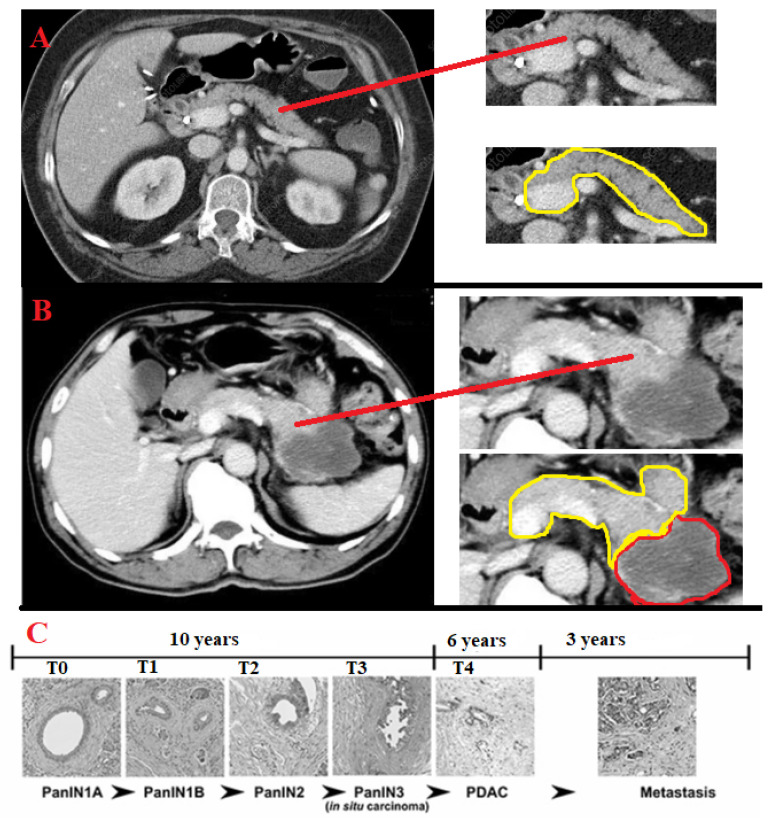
(A) A normal pancreas CT image; (B) PDAC at an advanced stage, with spreading to other organs; (C) Stages of PDAC development, which are represented as T0 to T4. The other stage, metastasis, is not represented because cancer at that point has spread to other organs surrounding the pancreas. PanIN (pancreatic intraepithelial neoplasia) with low-grade pancreatic intraepithelial neoplasia encompasses three older terms—PanIN-1A, PanIN-1B, and PanIN-2. These include Pancreatic Intraepithelial Neoplasia 1-A, Pancreatic Intraepithelial Neoplasia 1-B, and Pancreatic Intraepithelial Neoplasia 2. Pancreatic Intraepithelial Neoplasia 3 is high-grade pancreatic intraepithelial neoplasia, which then advances to PDAC.
